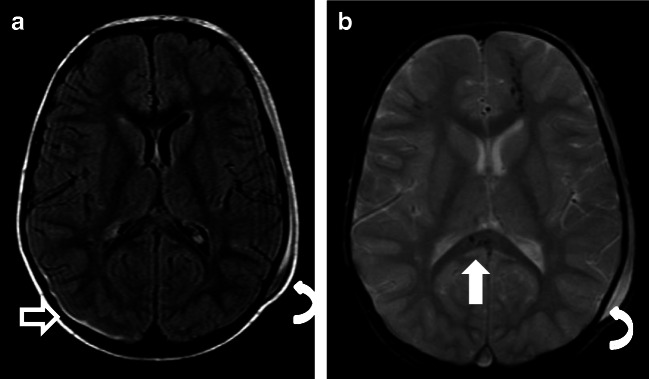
Case “NAHT2.” 2 years old. a FLAIR. b T2*. Anamnesis (not available for the neuro/radiologists): hit by a car as a pedestrian the day before. MRI findings: a coup injury with soft tissue edema on the left side (a and b, curved arrow) with a contrecoup on the opposite side due to abrupt deceleration of the head. The rapidly changing velocities within the skull may stretch and tear small bridging veins. Much more common than epidural hemorrhages, subdural hemorrhages (a, black straight arrow) generally result from shearing injuries due to various rotational or linear forces. Note on T2* the diffuse axonal injury (b, white straight arrow) with a characteristic distribution: typically located in the corpus callosum, white matter, and gray-white matter junction on the left frontal lobe. All neuroradiologists were able to rule out pAHT but none of the non-neuroradiologists
